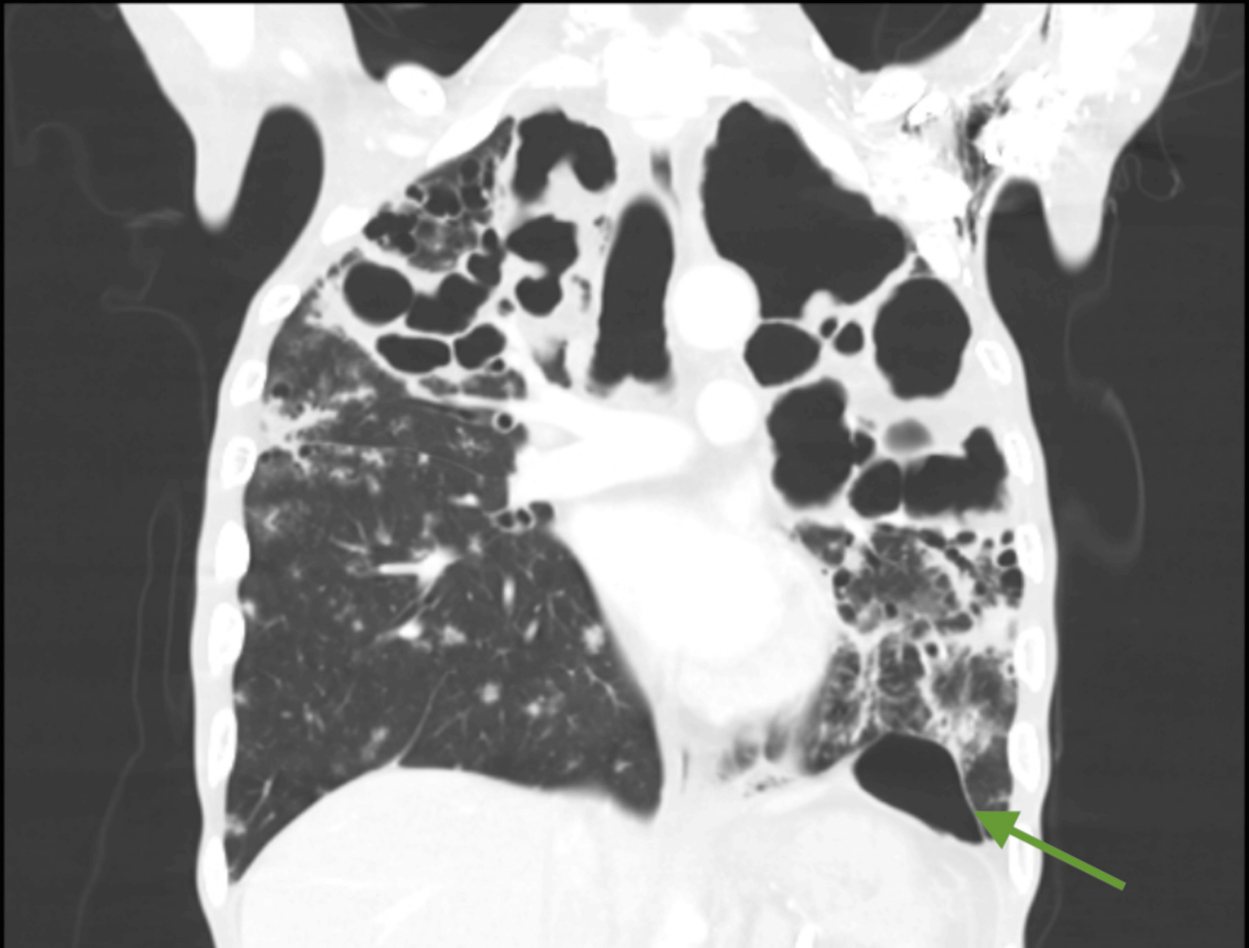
Coronal plane of the of chest on a contrast enhanced CT Extensive bilateral bronchopulmonary architectural distortion with multiple large, confluent cavitary lesions. The most affected regions included the right and left upper lobes, with the largest cavitation measuring approximately 8.5 × 7.7 cm. Green arrow: The left subpulmonic pneumothorax is visible between the heart and the diaphragm with the consolidation foci above it.
